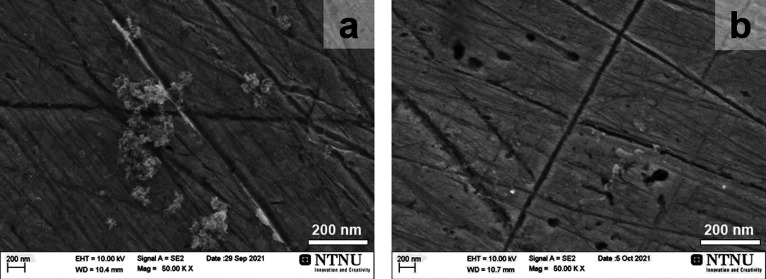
SEM images of (a) Ni(poly) electrode before ultrasonication
